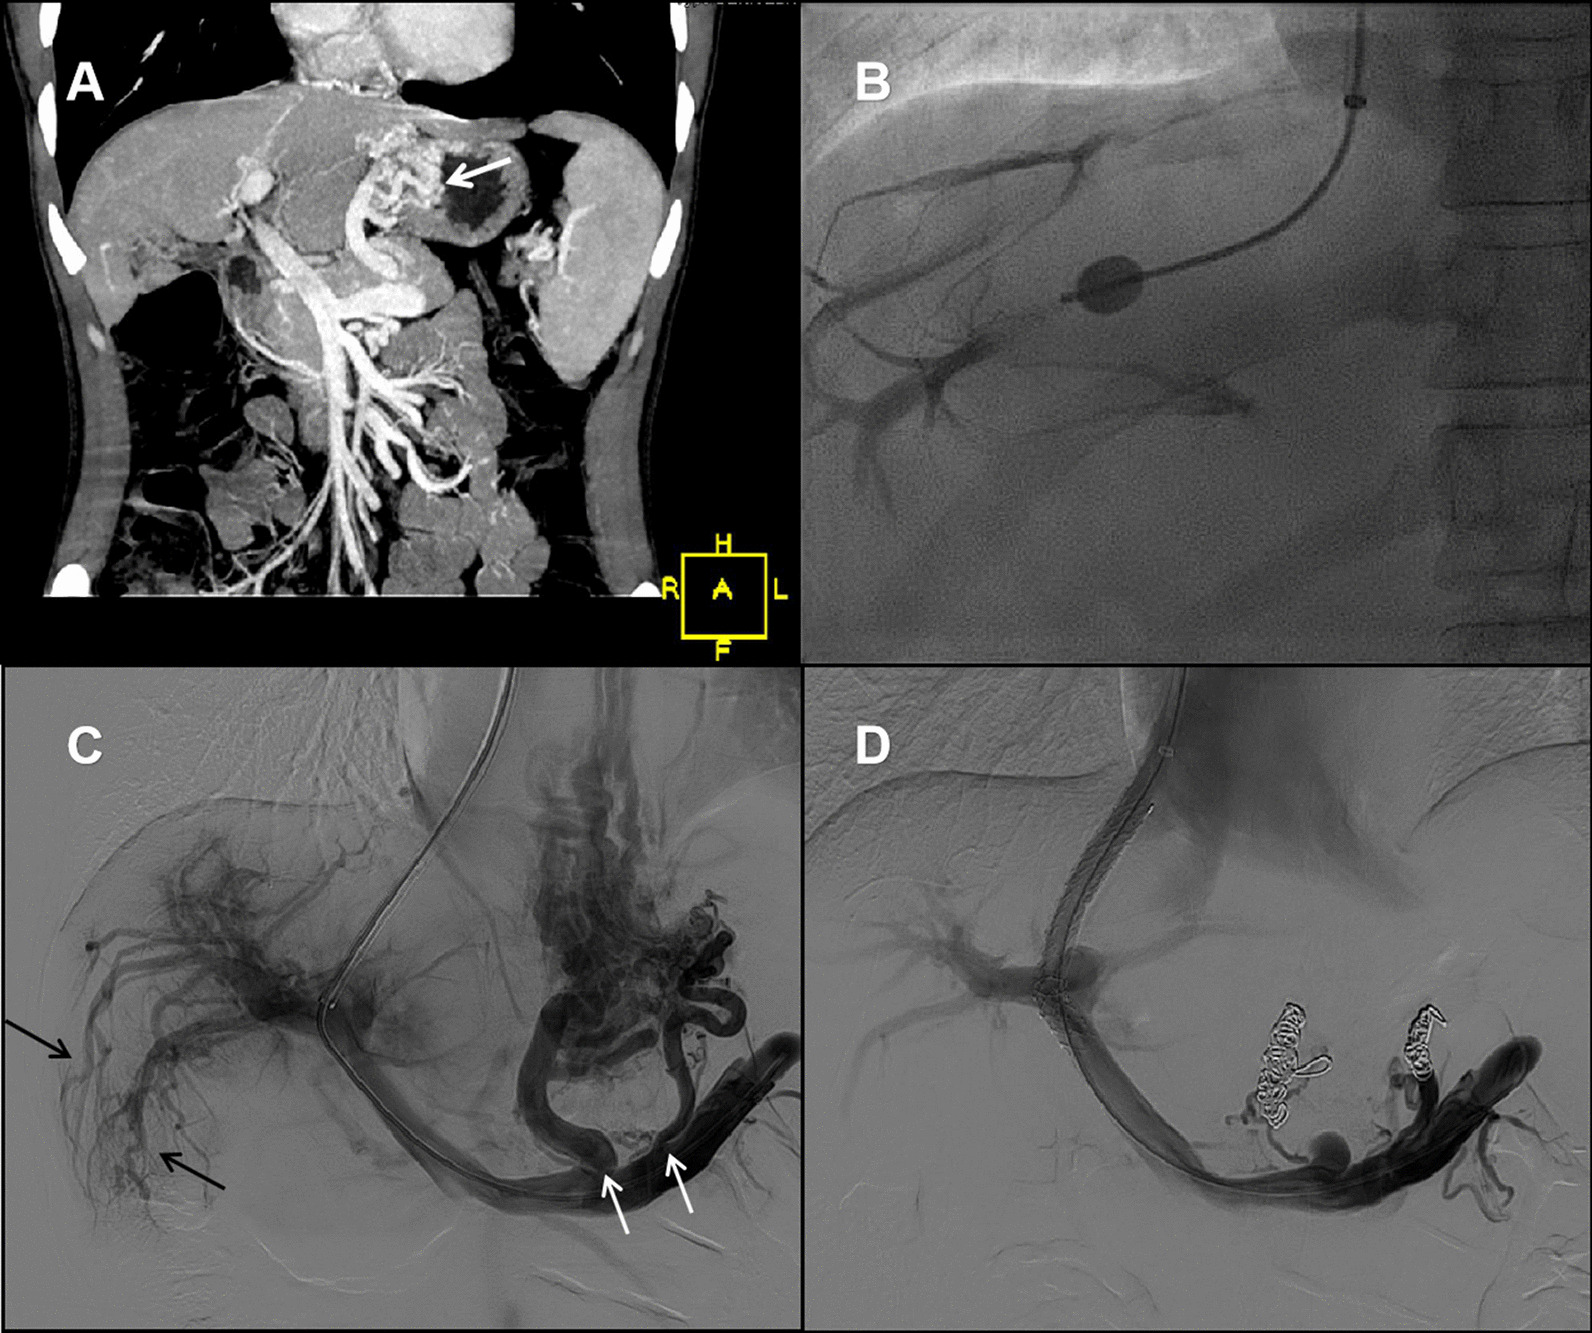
A Contrast-enhanced abdominal CT angiography with three-dimensional reconstruction showed a smooth liver surface, splenomegaly and gastroesophageal varices (white arrow). B Hepatic vein-to-vein communication was detected by hepatic venography. C Portography showed irregularity and tortuosity of the peripheral portal branches with much abrupt interruptions (black arrow) and avascular area beneath the liver surface. Portovenous collaterals (white arrows) derived from gastric coronary vein were also displayed. D Post-TIPS portography revealed embolization of collaterals and clear stent flow from portal vein to systemic circulation
